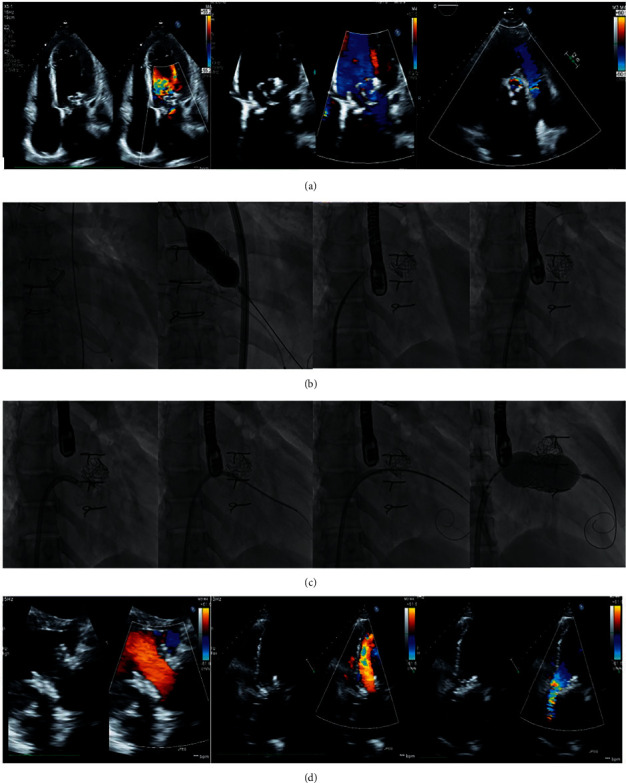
Transcatheter mitral valve in a degenerated EPIC™ (St. Jude Medical) valve (TMViV) with a concomitant transcatheter aortic valve implantation (TAVI). A 49-year-old old female patient with COPD had a degenerated bioprosthetic 29 mm EPIC™ MV and a degenerated bioprosthetic AV with an associated AF. TTE shows a degenerated bioprosthetic 29 mm EPIC™ MV (severe MS) and a degenerated bioprosthetic AV (severe AS). (b) Fluoroscopy shows the crossing of degenerated bioprosthetic AV with a 5F Amplatz left catheter over a 0.035-inch curved Terumo guidewire, followed by transaortic TAVI of an Edwards SAPIEN 3-26 mm valve during rapid pacing. Transseptal puncture using a 0-1 BRK™ within an 8.5F-SL sheath, followed by balloon dilatation of the transseptal puncture using a 12 mm balloon. (c) Fluoroscopy shows a transseptal TMViV of an Edwards SAPIEN-3 29-mm valve. First, an 8.5F-agilis™ sheath was flexed and directed towards the degenerated bioprosthetic MV. Second, a 5F-MP catheter over a 0.035-inch curved Terumo guidewire crossed the MV to the LV. Third, the wire was exchanged with a 0.035-inch/260 extra-stiff Confida™ guidewire. And finally, THV implantation. TTE: both SAPIEN-3 valves are in mitral and aortic positions with normal flow across both valves. AF: atrial fibrillation, AS: aortic stenosis, AV: aortic valve, COPD: chronic obstructive pulmonary disease, MS: mitral stenosis, MV: mitral valve, MVA: mitral valve area, Pg: pressure gradient, TAVI: transcatheter aortic valve implantation, TMViV: transcatheter mitral valve-in-valve, TTE: transthoracic echocardiography, and THV: transcatheter heart valve.
